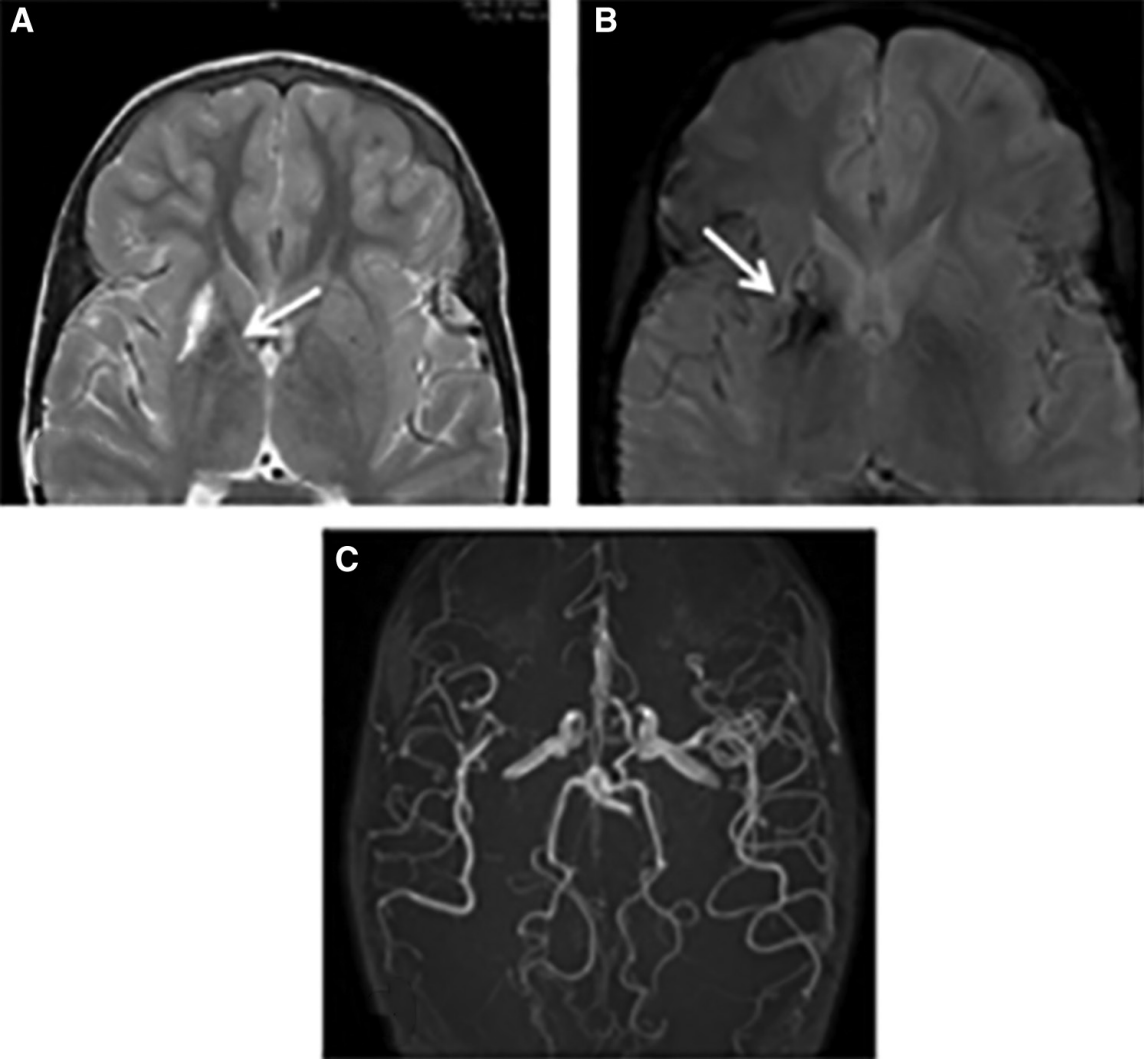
A, Hemorrhagic basal ganglia infarct in a 16-y-old girl not reporting symptoms but with asymmetric transcranial Doppler velocities (right time averaged mean of the maximum 39, left 164 cm/sec). The subtle hemorrhagic change is seen as a dark blush on the T2-weighted sequence but is well seen on the (B) T2* sequence (white arrows). C, A magnetic resonance angiogram revealed severe stenosis of the right middle cerebral artery with reduced filling of the distal vessels. She was followed for 3 y and did not develop neurological symptoms.
